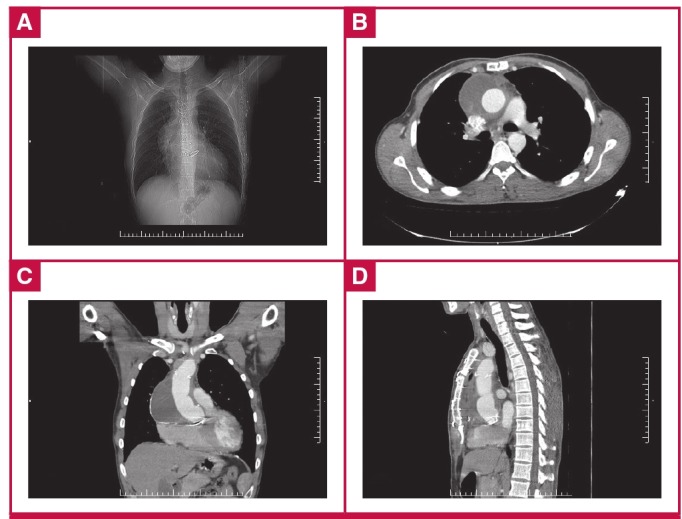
Postoperative radiography (A). Postoperative thoracic CT scan: axial (B), coronal (C) and sagittal reconstructions - mediastinal window view (D).
